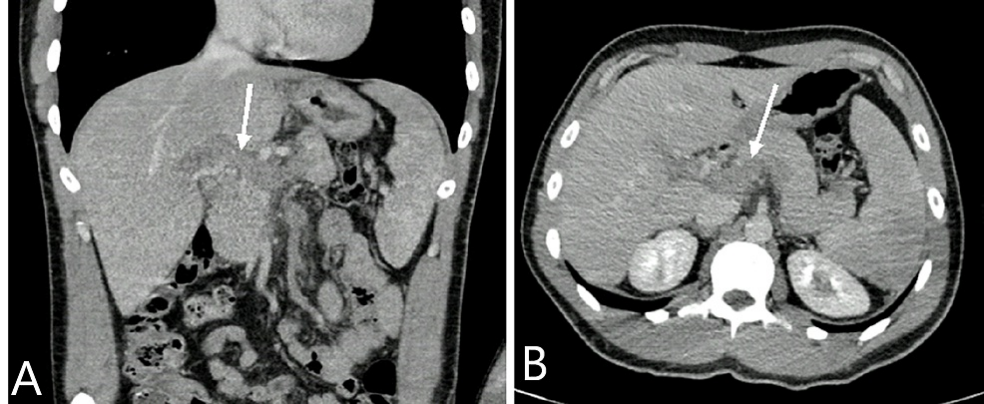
Thoracoabdominal CT scan showing portal mesenteric venous thrombosis (arrows) A: coronal view, B: axial view
